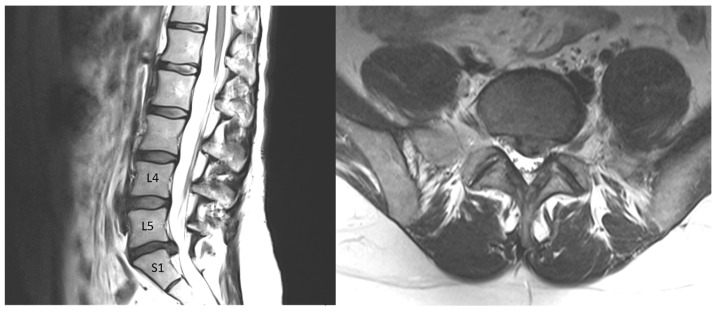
T2-weighted images in sagittal and axial projections. At the L4/5 level, control disc, and at the L5/S1 level, visible left-sided protrusion of dehydrated disc.
